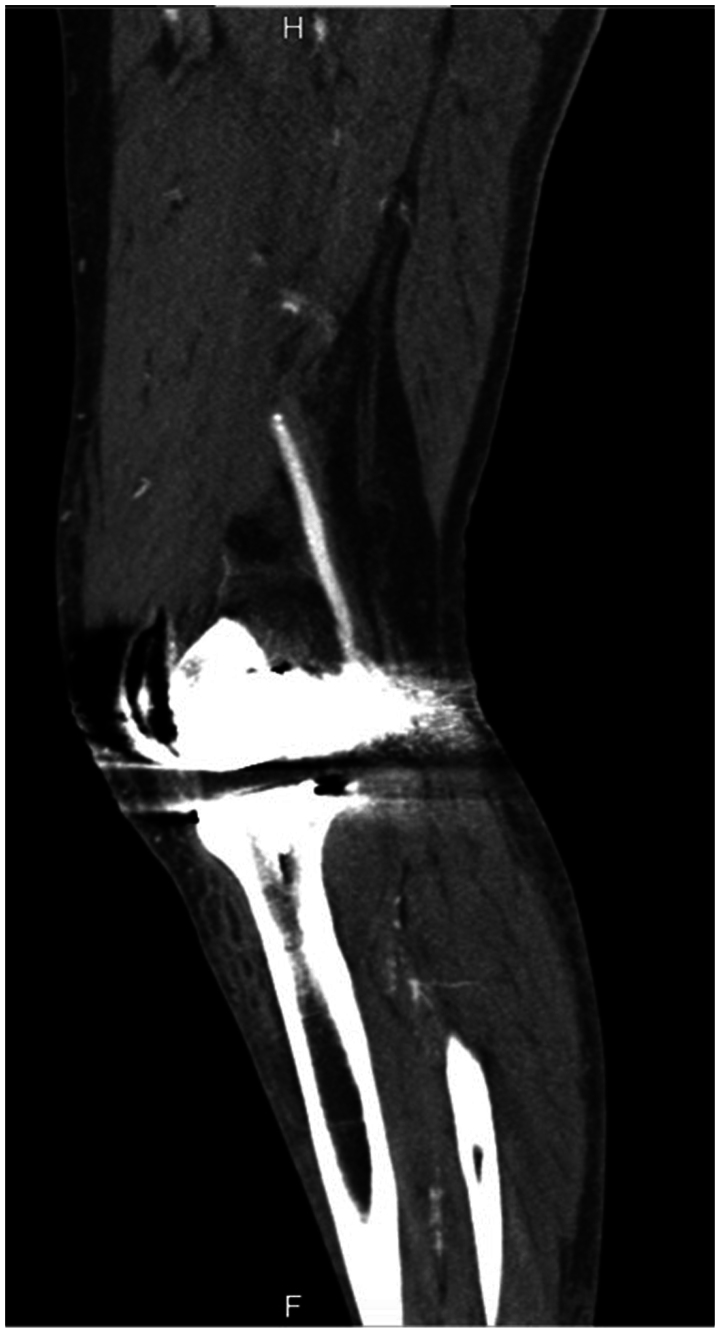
Computed tomography image following the total knee arthroplasty (TKA) showing the occlusion of popliteal artery (PA).
